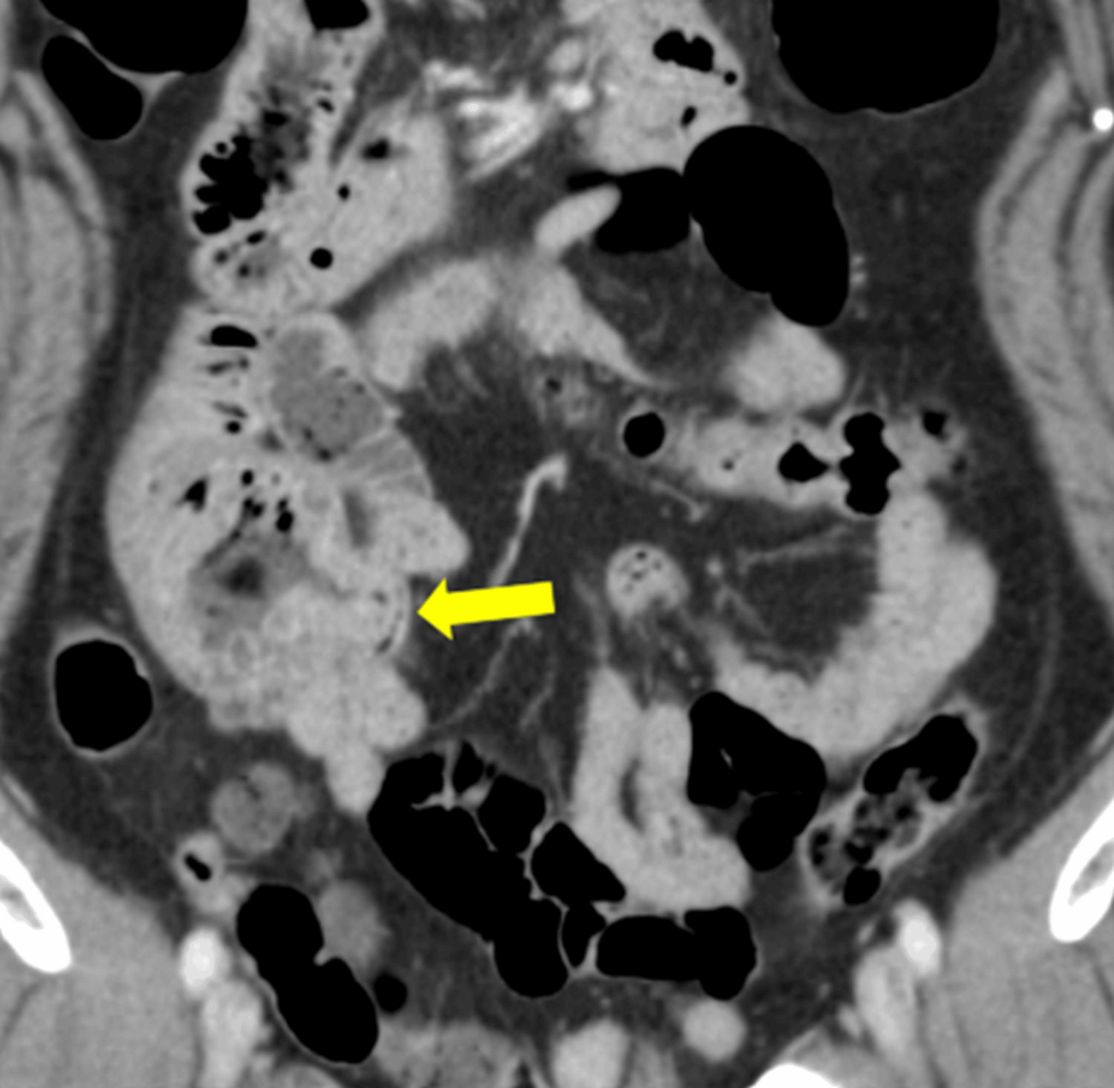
Abdominal CT Abdominal CT showed wall thickening of the jejunum. CT: computed tomography
